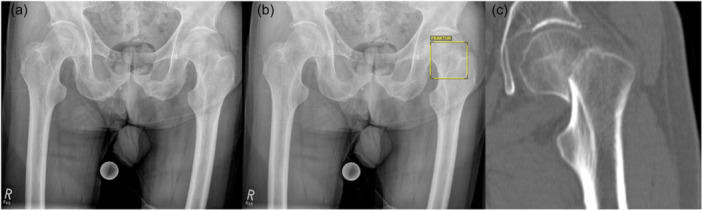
(a) Anteroposterior pelvic radiograph with example of a non‐displaced Femoral neck fracture initially not described in the radiological report. (b) Artificial Intelligence correctly identifying the fracture. (c) Confirmatory CT scan. CT, computed tomography.
